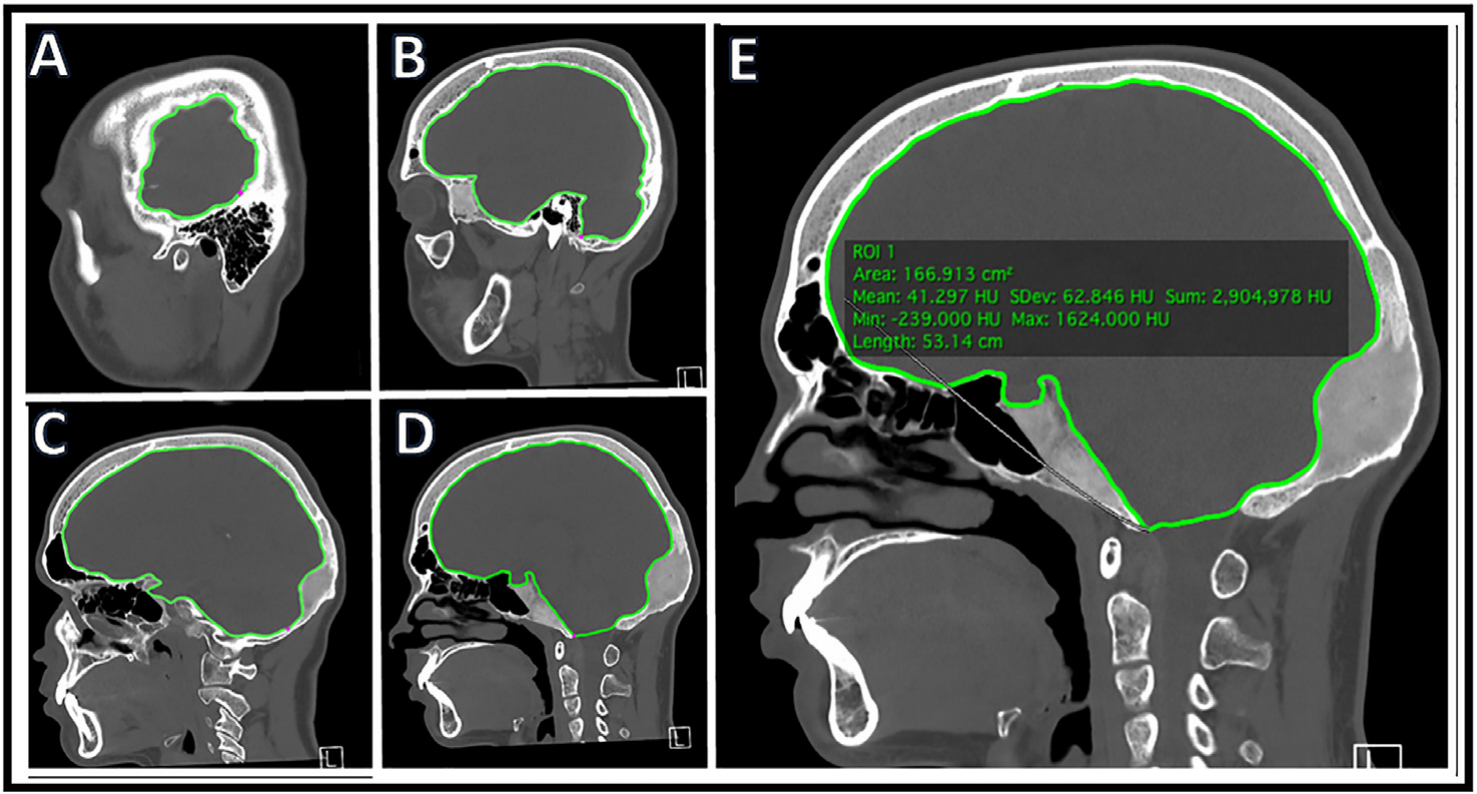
Craniomorphometric analysis of intracranial volume in a subject with fibrous dysplasia/McCune-Albright syndrome, utilizing OSIRIX software. Using CT head imaging with ≤5 mm axial slices, a region of interest (ROI) was traced to include intracranial area. Tracing was started with lateral slices (A, B) and advanced towards medial slices (C-E). The software calculated the area encompassed within ROIs and used this to determine total volume (E).
